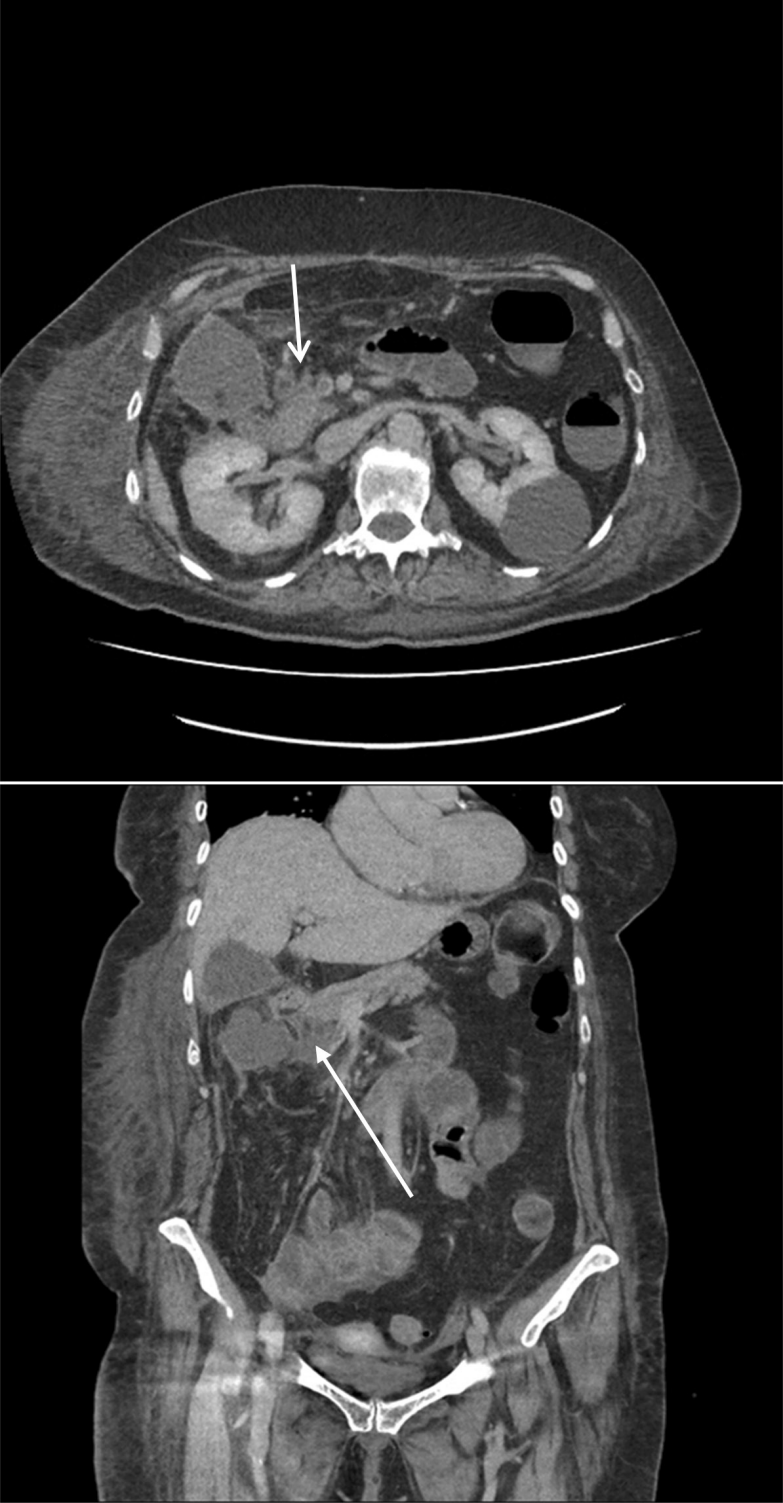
Postoperative CT scan of the abdomen on 12/02/2021: Thrombosed right‐sided tributary of the superior mesenteric vein (SMV).
